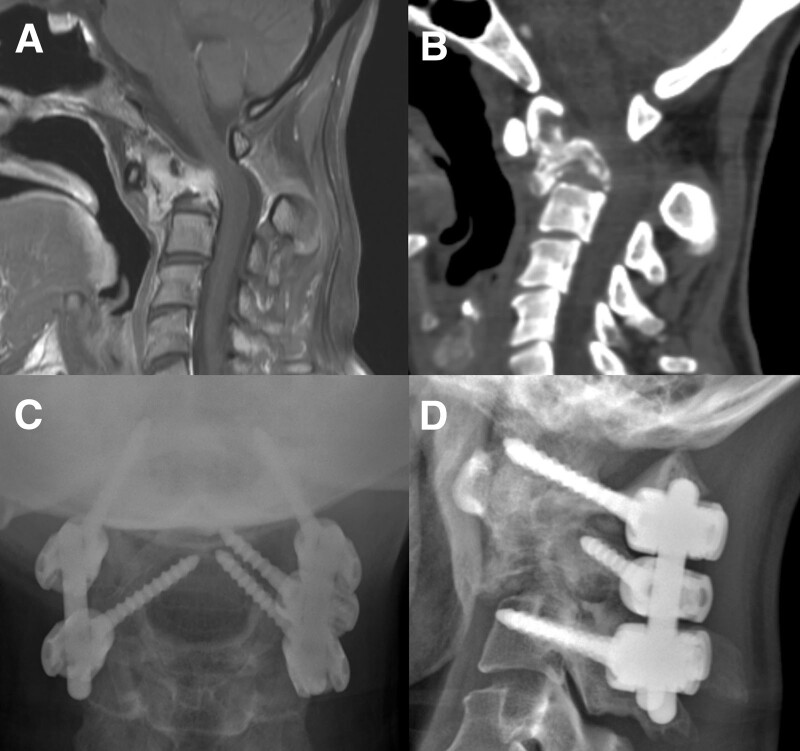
A 44-year-old woman diagnosed with breast cancer presented to the clinic with neck pain and a tingling sensation in both arms. Initial MRI (A) reveals a collapse of the C2 body with a metastatic tumor, and CT (B) shows an osteolytic tumor at the C2 body. Occiput-preserving CPS is performed from C1 to C3. Postoperative AP (C) and lateral (D) X-ray films show well-fixed pedicle screws. AP = anteroposterior, CPS = cervical pedicle screw, CT = computed tomography, MRI = magnetic resonance image.
